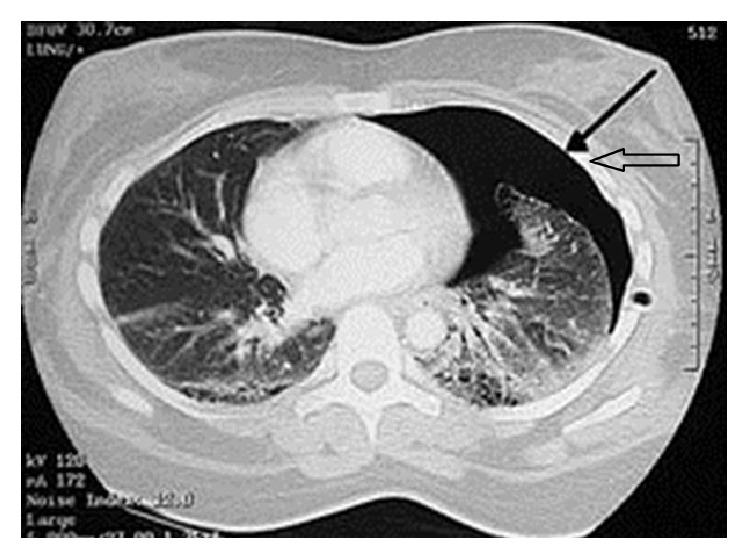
Chest CT scan showing left-sided pneumothorax.
